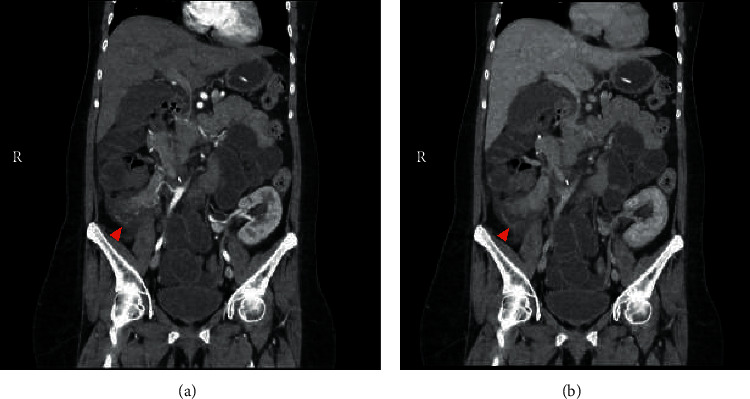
Computed tomography of the abdomen in coronal view. (a) Arterial phase demonstrates satisfactory enhancement of pancreatic artery (red arrow). (b) Venous phase demonstrates poor enhancement of pancreatic tail with surrounding inflammatory changes (red arrow).
